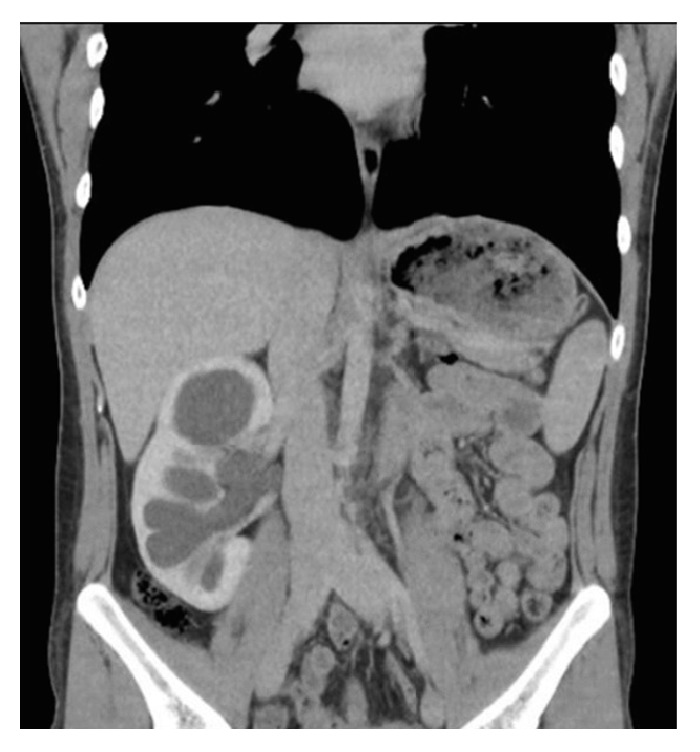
UPJO in solitary kidney with intrarenal pelvis in urography images by CT scan.
